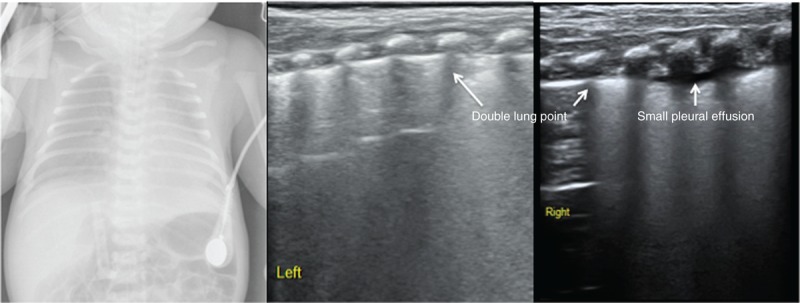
Differentiation between TTN and RDS. The infant above had a GA of 38 wk and was delivered by cesarean section; the infant had a birth weight of 2750 g. The infant started to experience progressive dyspnea soon after birth. The infant was diagnosed with RDS based on medical history and clinical and CXR manifestations. However, lung ultrasonography only showed pleural line abnormalities, white lung, pleural effusion, and A-line disappearance without lung consolidation. Therefore, the infant suffered from TTN rather than RDS. The clinical course of the infant was also consistent with TTN. CXR = chest radiography, GA = gestational age, RDS = respiratory distress syndrome, TTN = transient tachypnea of the newborn.
